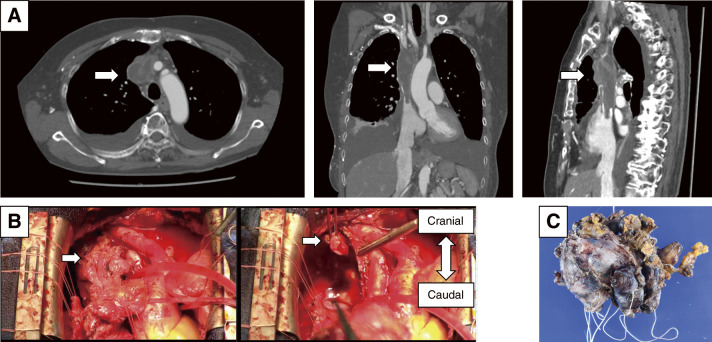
Surgical findings of invasive thymic carcinoma. (Patient No. 2) Contrast-enhanced computed tomography revealed an anterior mediastinal mass and poor opacification of the SVC, which indicated thrombus or bland thrombus (panel A, arrows). Intraoperative findings are shown in panel B. Median sternotomy was performed, and the anterior mediastinal tumor was carefully dissected. Cardiopulmonary bypass was established via the right femoral artery and right atrium. The tumor within the SVC was completely adherent along its full length (left image; arrow indicates the tumor). The SVC was divided proximally (right image; arrow indicates the SVC). The tumor was resected en bloc (panel C). The right phrenic nerve was involved. Reconstruction of the SVC was performed using a PTFE graft. SVC, superior vena cava; PTFE, polytetrafluoroethylene
